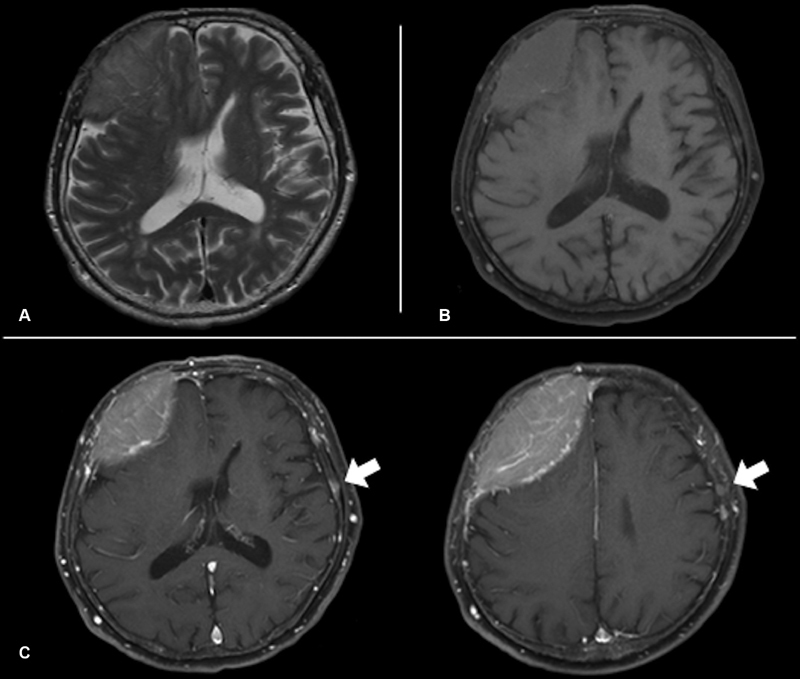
Magnetic resonance imaging on admission. The mass showed iso-signal intensity in T1 (
